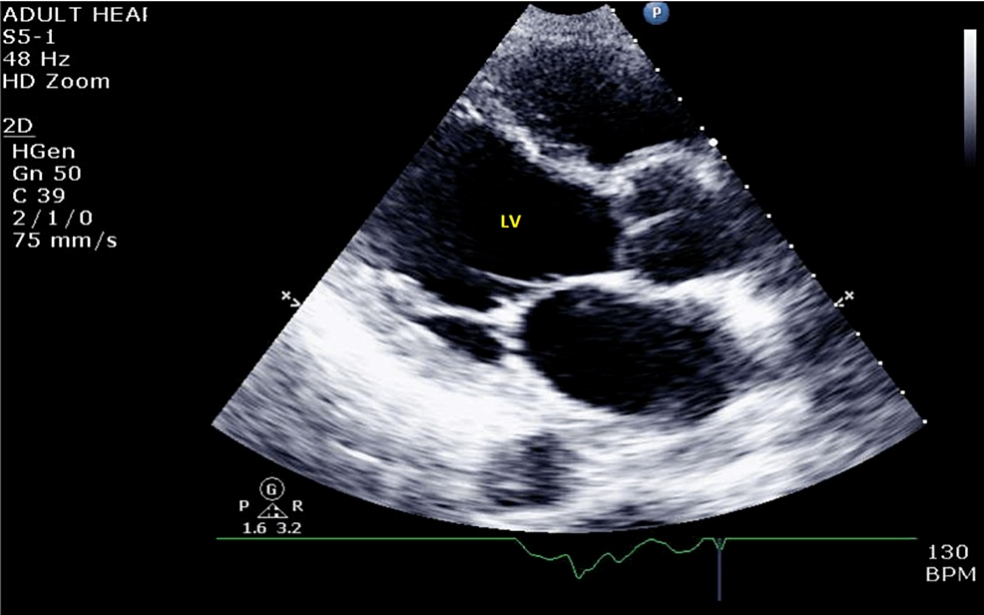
Parasternal long view of the dilated left ventricle on echocardiography. LV: left ventricle
